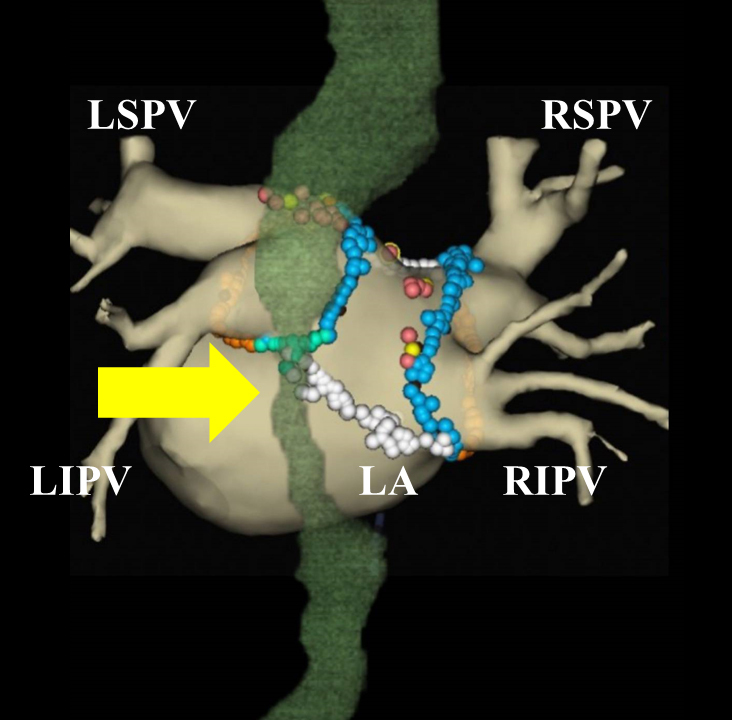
Three-dimensional computed tomography revealed that the patient’s esophagus (green) was tapered and became hypercontractile like a jackhammer (yellow arrows). The colored tags indicate ablation lines. LA = left atrium, LSPV = left superior pulmonary vein, LAPV = left inferior pulmonary vein, RSPV = right superior pulmonary vein, RIPV = right inferior pulmonary vein.
